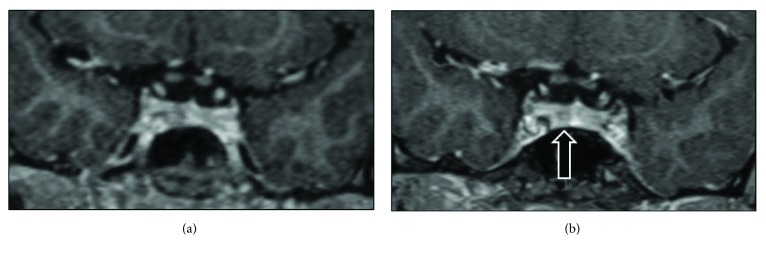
Pituitary MRI scans of the proband's first cousin (case 5), showing a normal pituitary at the age of 9 (a), and three years later, a 3 mm microadenoma became visible in her pituitary gland (b).
